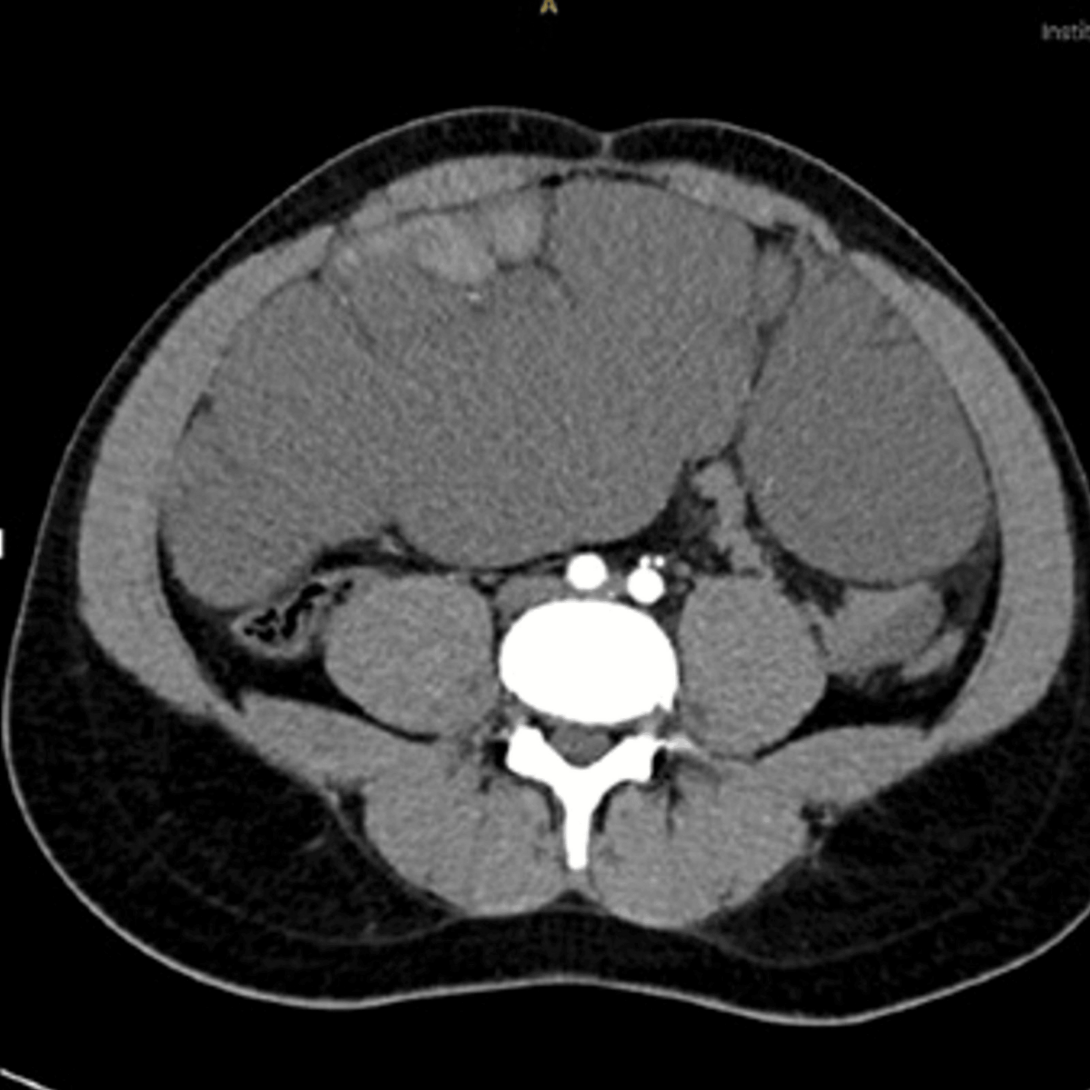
Abdominal computed tomography (CT) showing multiloculated cystic structures filling the entire omentum, and displacing the bowel loops posteriorly
